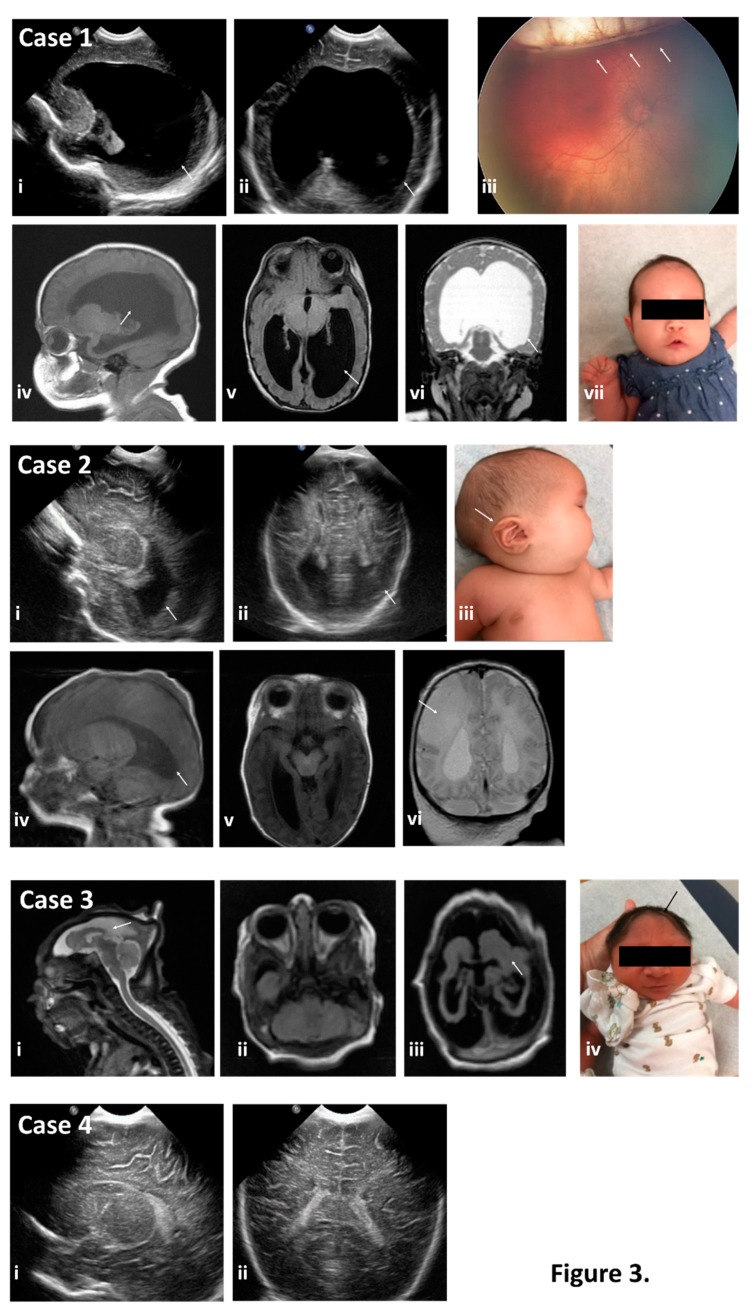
Neonatal physical exam findings consistent with a diagnosis of congenital Zika syndrome as identified by neonatal head ultrasound, postnatal MRI, gross presentation, and ophthalmic examination for the cases where detailed examination was warranted and subjects returned for postnatal care. Case 1 exhibited severe ventriculomegaly (arrows in images i and ii) by neonatal head ultrasound and as confirmed by post-natal MRI (arrows in images iv, v, and vi), despite the fronto-occipital head circumference (FOC) being normal (54th percentile) (image vii). Ophthalmic examination revealed bilateral colobomatous lesions consistent with congenital Zika syndrome (arrow in image iii). Case 2 exhibited colpocephaly of the lateral ventricles by head sonography (arrows in images i and ii), and postnatal MRI confirmed the colpocephaly (arrow in image iv) as well as callosal dysgenesis. Subject had low-set ears on exam (arrow in image iii) and a fronto-occipital circumference (FOC) at the 96th percentile with a cleft palate, microopthalmia, and asymmetric hypertonia with asymmetric EEG findings consistent with abnormalities in the right hemisphere. She developed neonatal seizures and failed her newborn hearing screen. Consistent with her clinical exam, she had findings concerning for an age-indeterminate vascular insult in the right middle cerebral artery territory with loss of gray-white differentiation and sulcal effacement within the right parietal lobe (arrow in image vi). Case 3 demonstrated significant cerebral dysplasia with severe microcephaly and supratentorial volume loss (arrows in images i, ii, and iii) on brain MRI. Physical exam also showed severe microcephaly (arrow in image iv) with FOC < −3.72 SD (<0.01%) per WHO growth curves. Case 4 exhibited no abnormal findings on postnatal head sonogram (images I and ii). Subject’s FOC was normal at the 36th percentile. No MRI in Case 4 was obtained given normalcy of physical exam and prenatal and postnatal sonographic findings.
